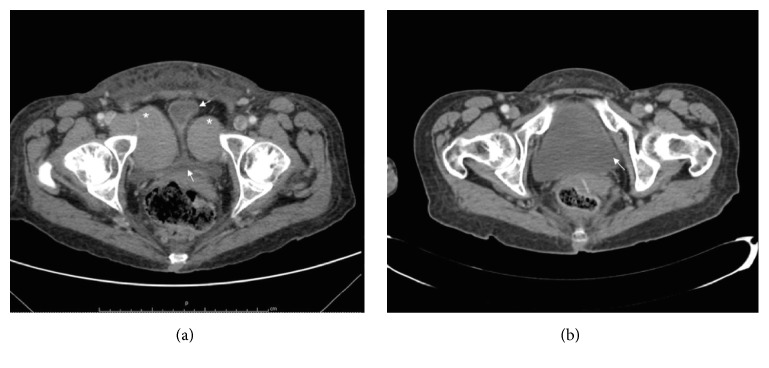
Pelvic CT before treatment with rituximab plus idelalisib (a). Hourglass deformity of urinary bladder (arrows) was seen as a result of the compression of two extraperitoneal paravesical soft tissue bulky masses (asterisks). After three months of idelalisib (b) a complete resolution of the masses was observer with a normal bladder.
